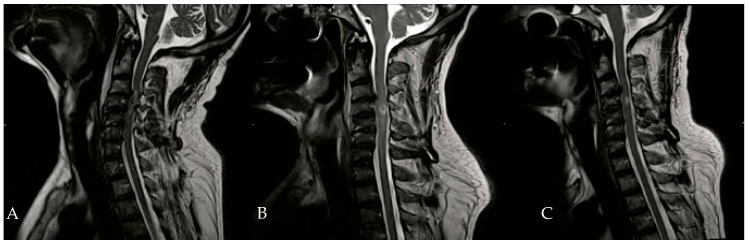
Evolution of hematomyelia in cervical MRI, performed 5 days (B) and 30 days (C) after the initial MRI (A).
